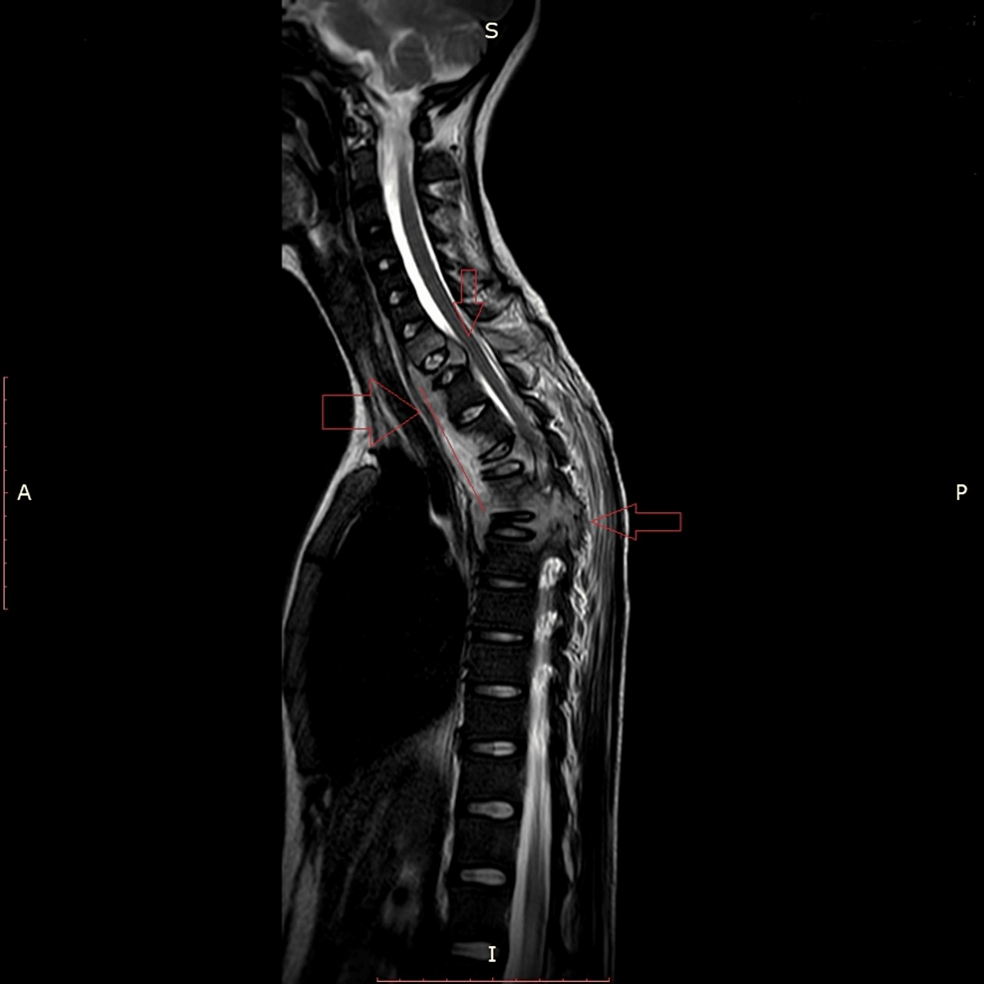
MRI cervical dorsal spine T2W shows altered intensities in the C7, D1, D3, D4, D5, and D6 vertebral bodies and the posterior elements of some of these vertebrae with compression collapse of the D1, D4, and D6 vertebral bodies.
